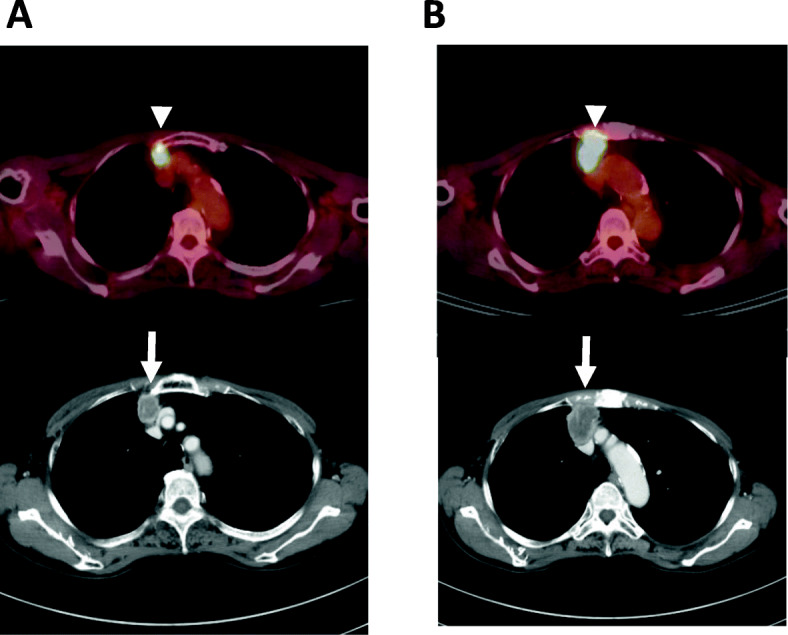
Imaging of ^18^F-FDG-PET in 74-years old female with advanced pulmonary adenocarcinoma who was treated with nivolumab monotherapy as 3rd line treatment. a PET imaging displayed the increased accumulation of ^18^F-FDG with SUV_max_ of 5.2 (white arrowhead) in the anterior mediastinal lymph node metastasis corresponding to CT (white arrow). b At 1 month after nivolumab initiation, the growth of the lymph node metastasis was observed on CT scan (white arrow) with increased uptake of ^18^F-FDG with SUV_max_ of 11.3 (white arrowhead)
